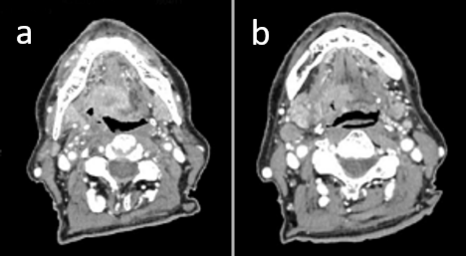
Constrast-enhanced CT scan of primary and neck.
